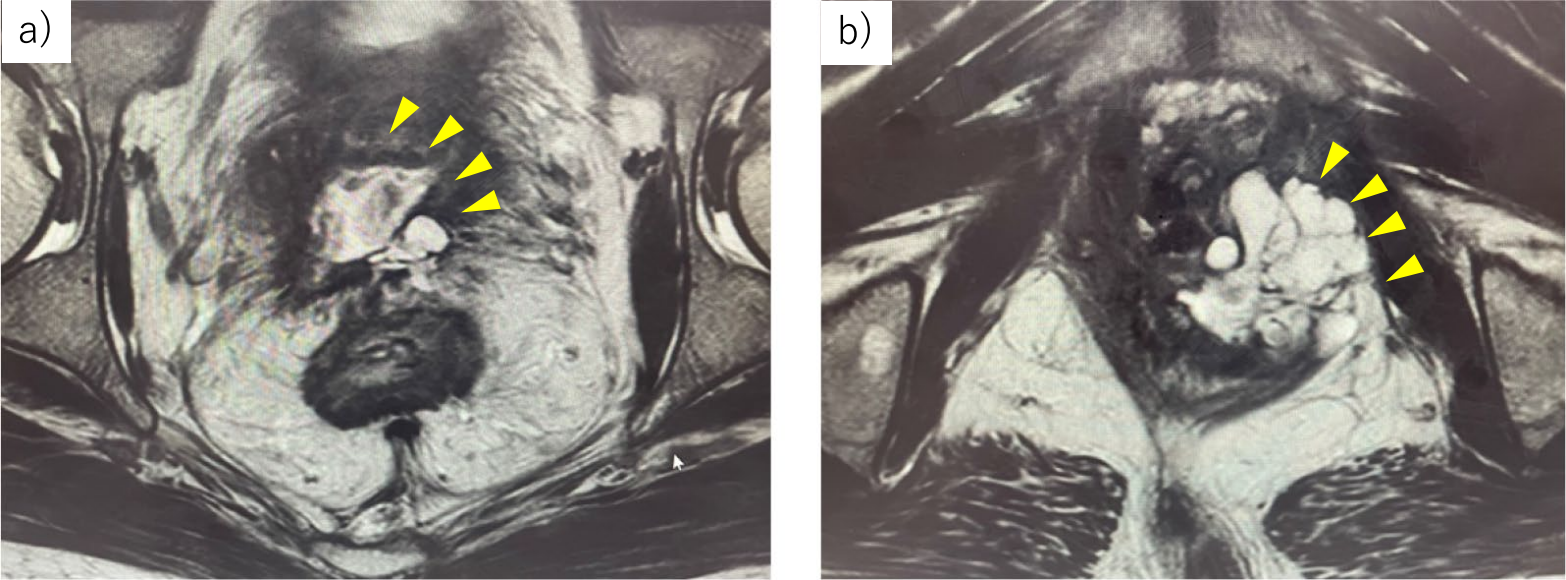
T2-weighted axial magnetic resonance images of the pelvis a The tumor, including a mucin pool, had spread to the prostate (yellow arrowheads). b Beyond the levator ani muscle, the tumor had invaded the obturator internus muscle on the left side (yellow arrowheads)
